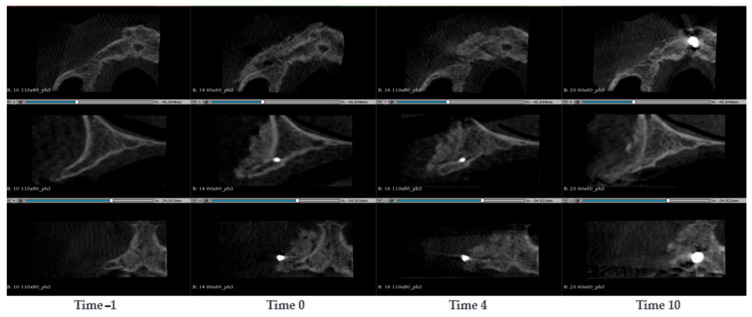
CBCT images of a 73-year-old woman with multiple devitalized teeth and bone resorption in the maxilla. A large SmartBone^®^ plate was fixed to the right-side maxilla. The images show the progression of bone healing over time, from pre-surgery (Time −1) to 10 months post-surgery (Time 10).
